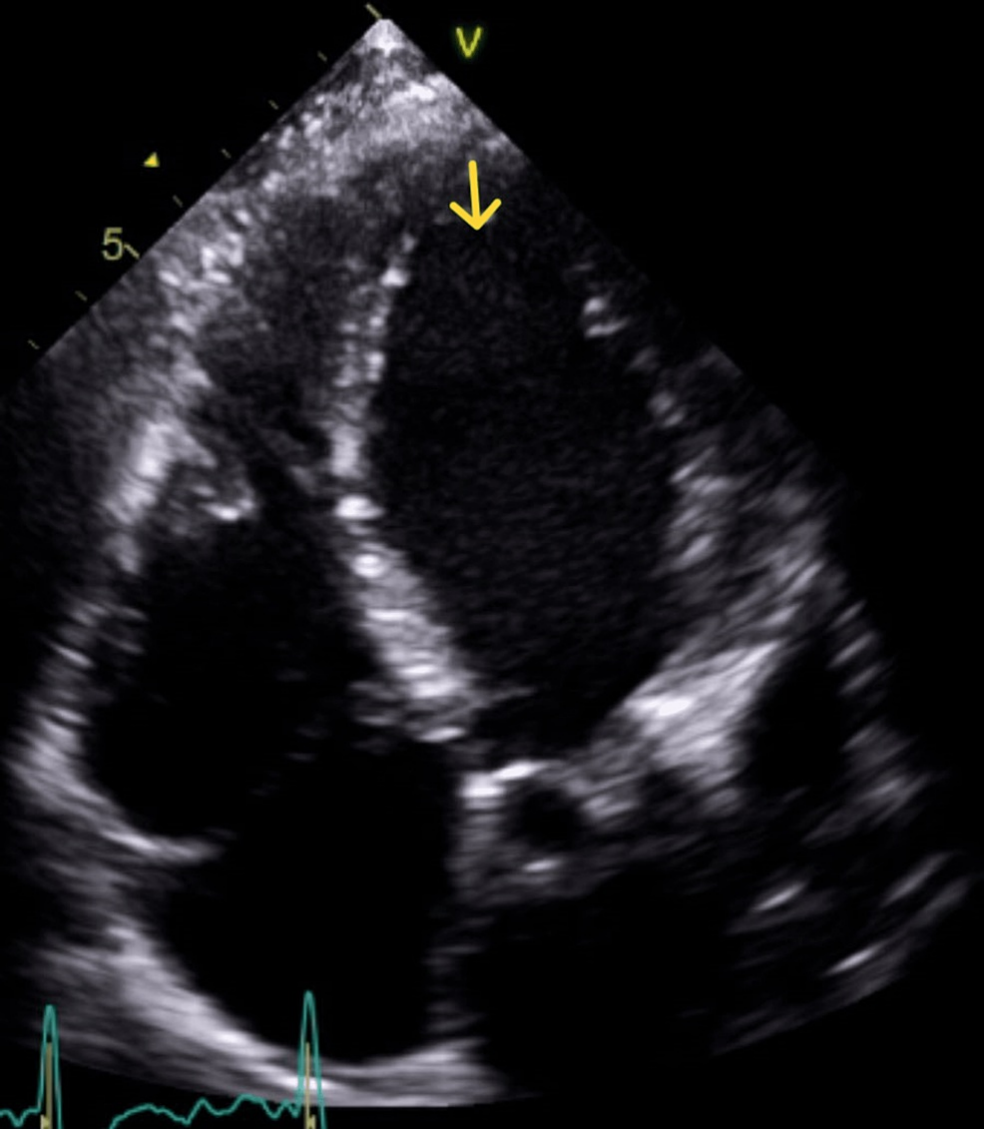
A repeat echocardiogram showing the resolution of thrombus
